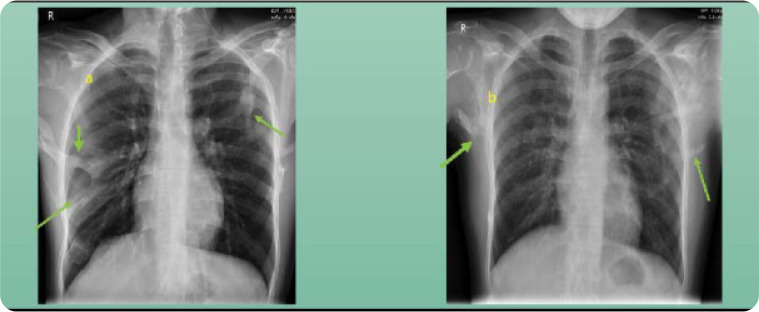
Chest radiographs from both the patients. Arrows pointing towards regions of heterotopic ossifications.
